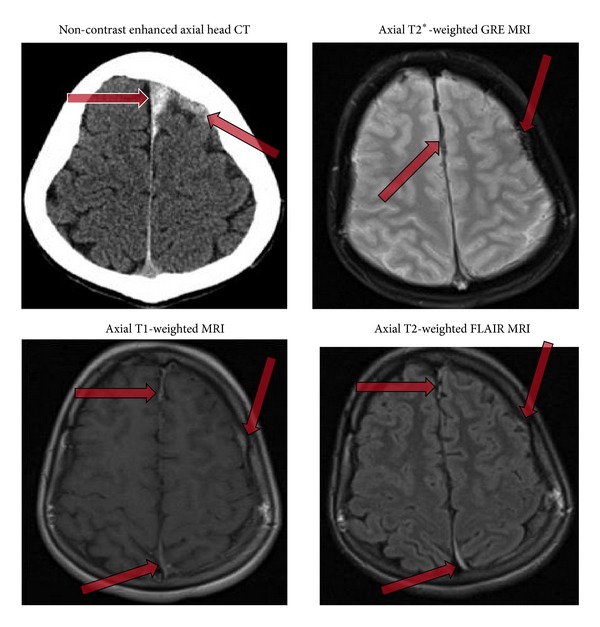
Axial noncontrast CT and MRI of the brain showing evidence of a thin (approximately 2-3 mm) subdural hematoma (arrows) over the left hemisphere with extension into the interhemispheric fissure.
